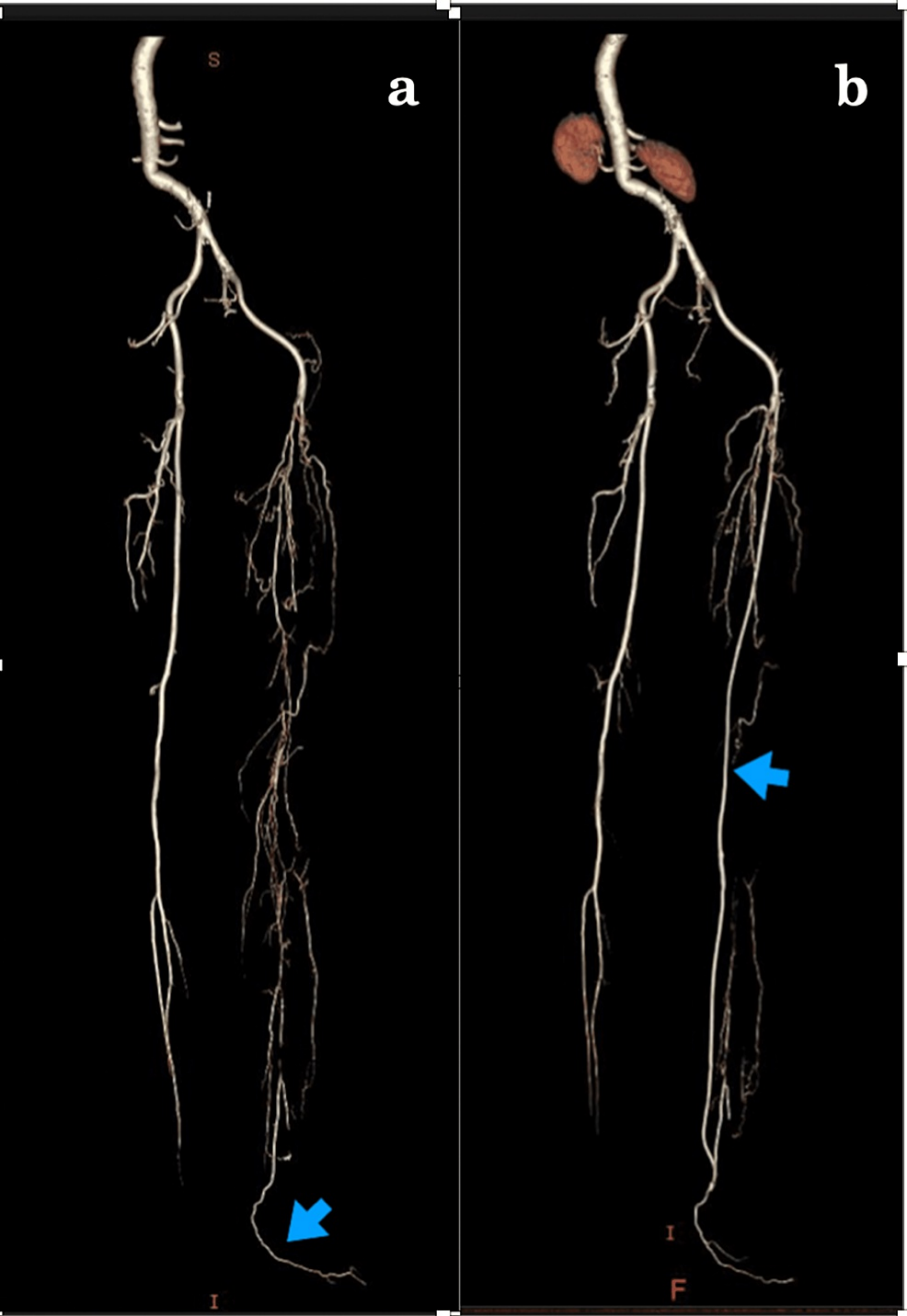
(a) Computed tomography angiography showing a long-segment occlusion of superficial femoral, popliteal, and tibial arteries, with distal reformation in a large ankle collateral (arrow). (b) Computed tomography angiography at the one-year follow-up showing patent vein bypass (arrow).
